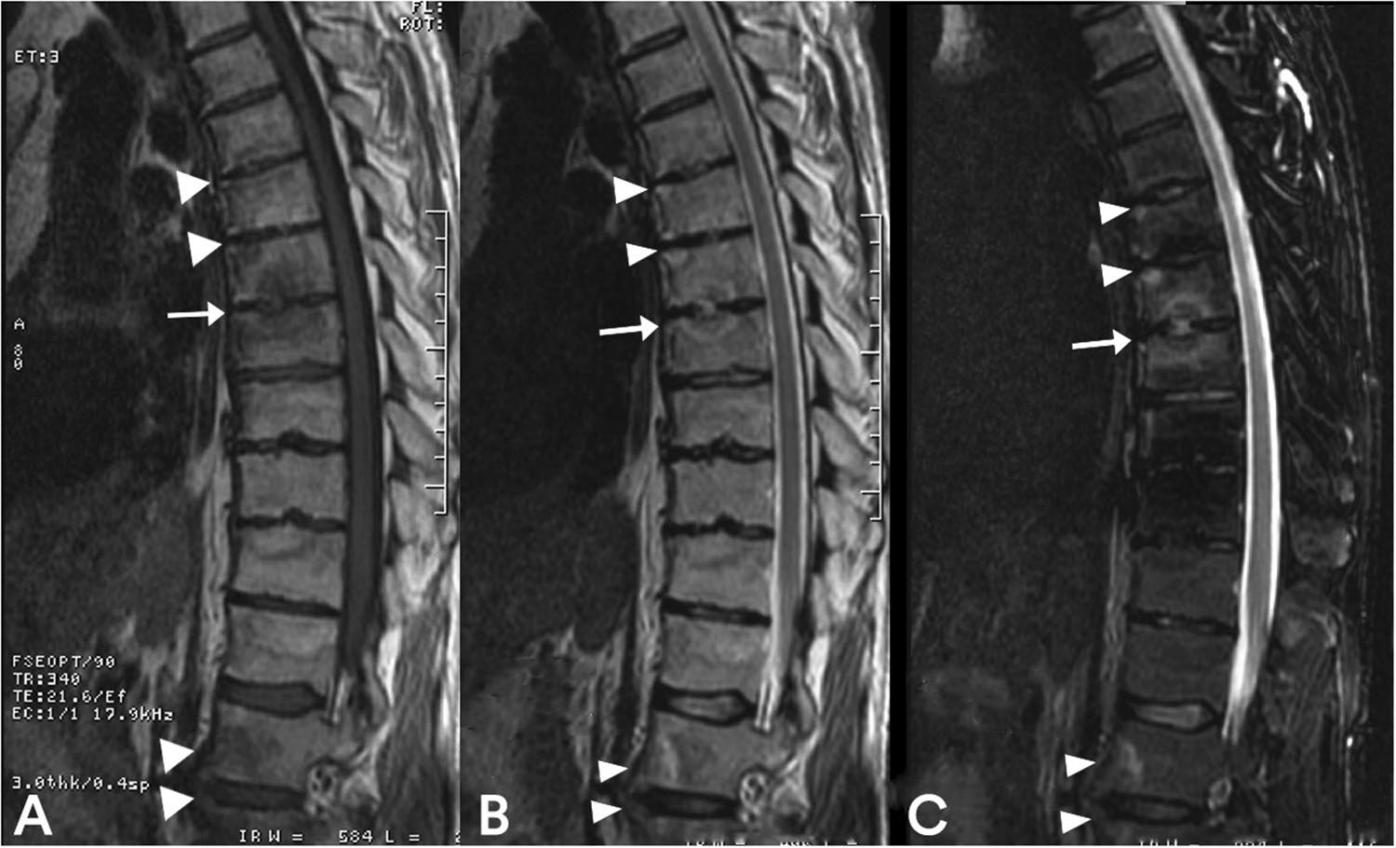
Sagittal T1-weighted image (a), sagittal T2-weighted image (b) and sagittal STIR image (c) showing a typical active Andersson lesion at D7/D8, with subchondral edema-osteitis, discitis and erosions (arrow). Romanus lesions can be observed in the anterior corners of the vertebral bodies at D6, D7 and at L1 and L2 (arrowheads)
